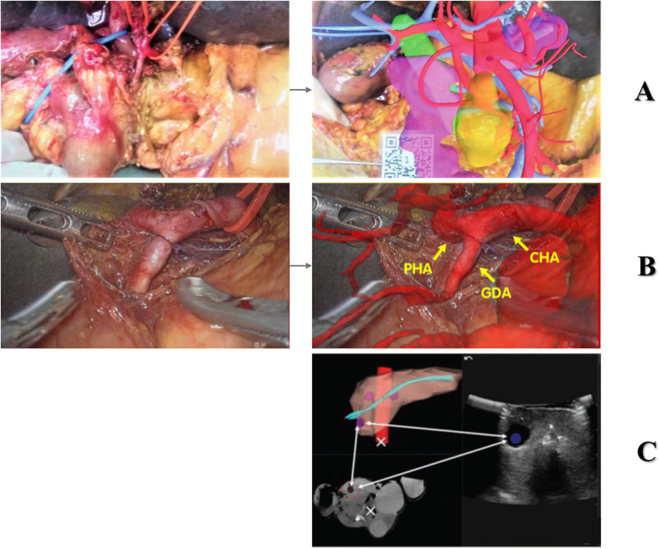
(A) Intraoperative view of surgical field and model registration using a QR code (image obtained from^[72]^); (B) intraoperative view of surgical field and model registration using anatomical markers (image obtained from^[76]^); (C) Landmark identification in a CT scan, ultrasound and 3D model (image obtained from^[77]^).
